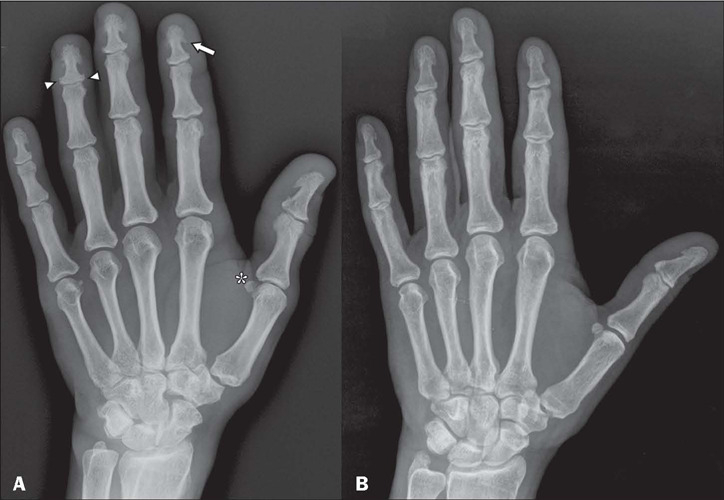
A: Conventional radiograph, in an anteroposterior view, of the right
